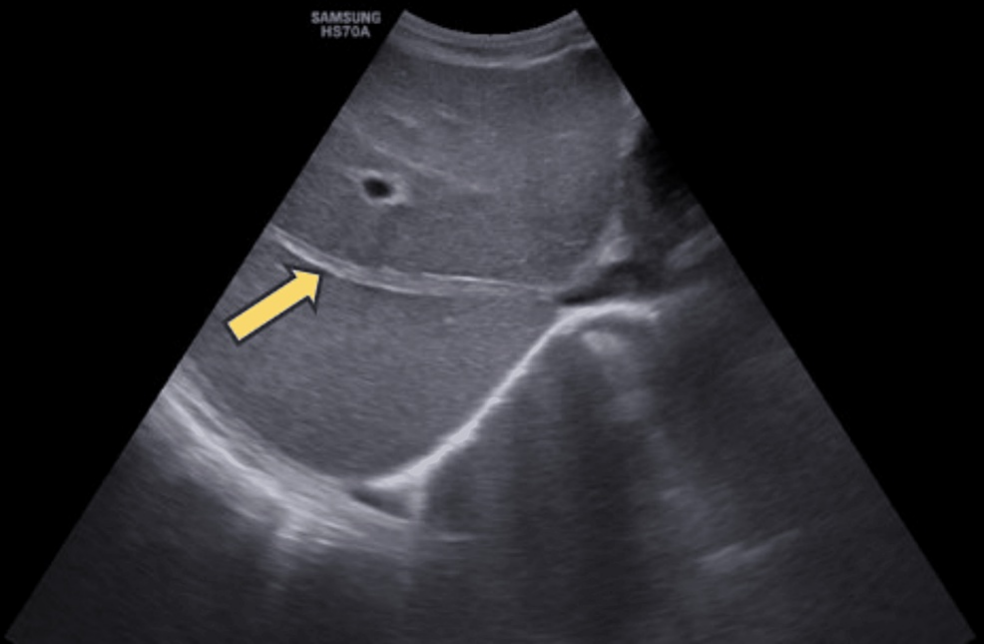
Grayscale ultrasound showing a fibrous cord replacing the middle hepatic vein (yellow arrow)
